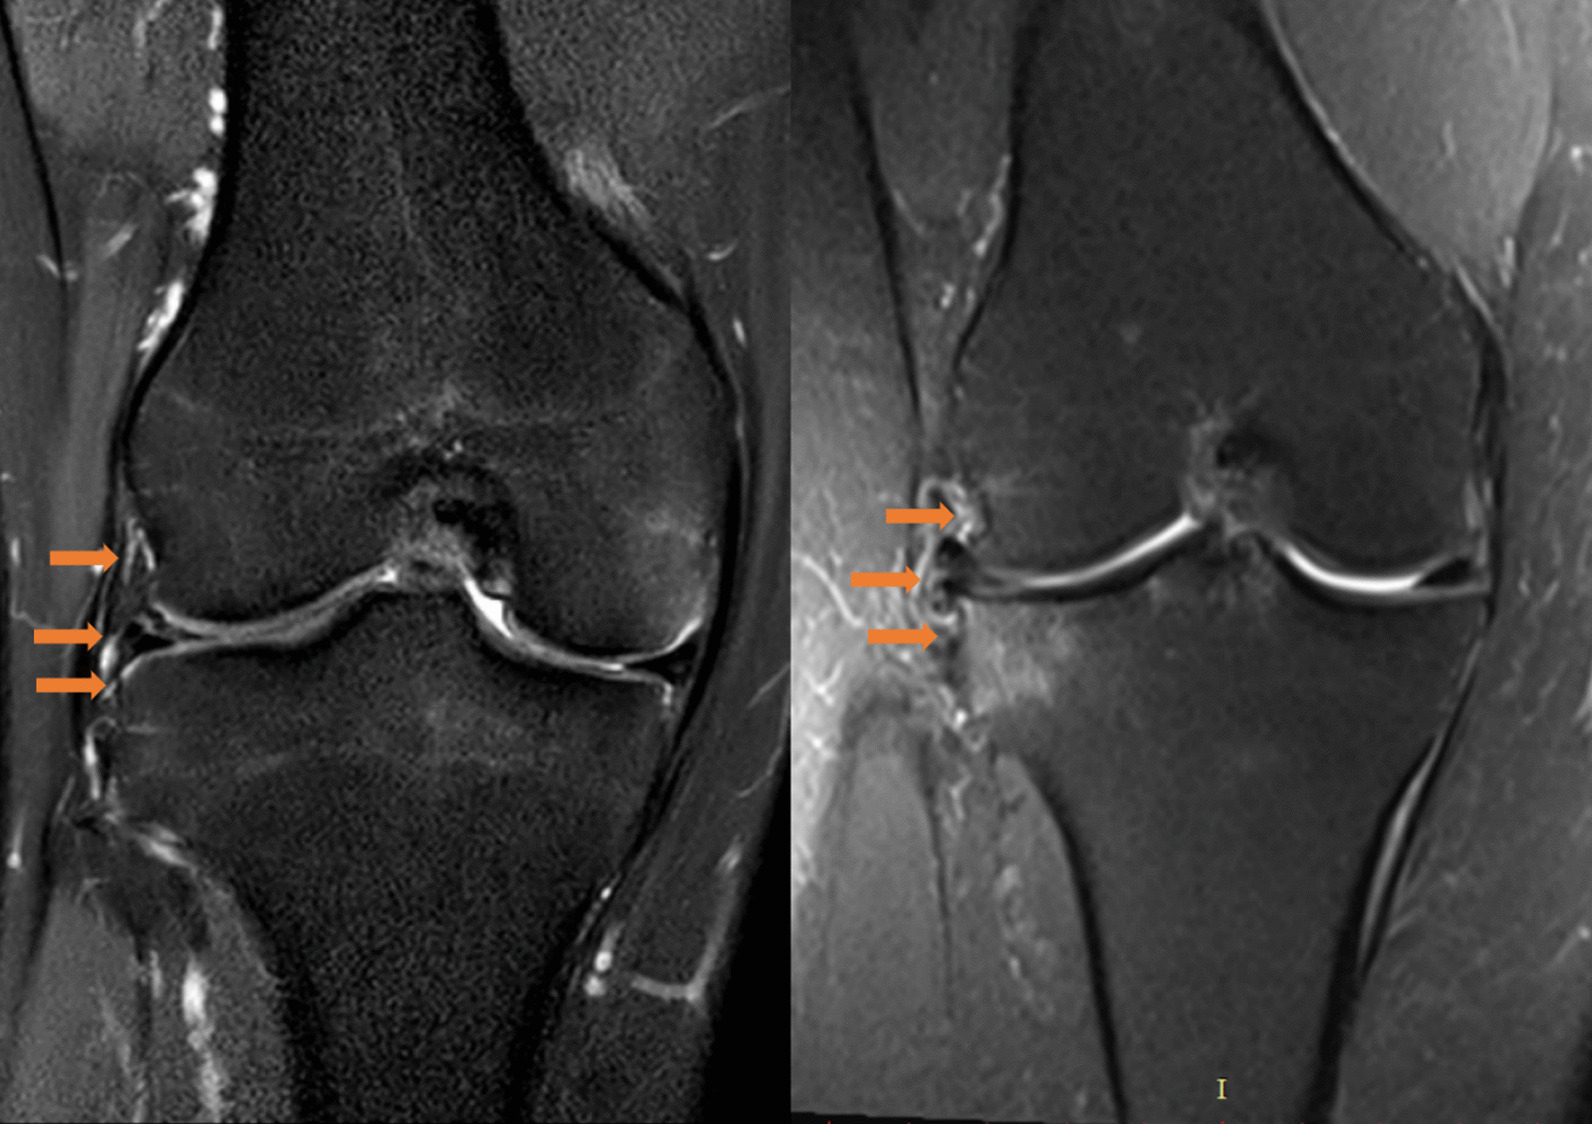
Representative magnetic resonance images of normal and abnormal ALL. ALL, anterolateral ligament
